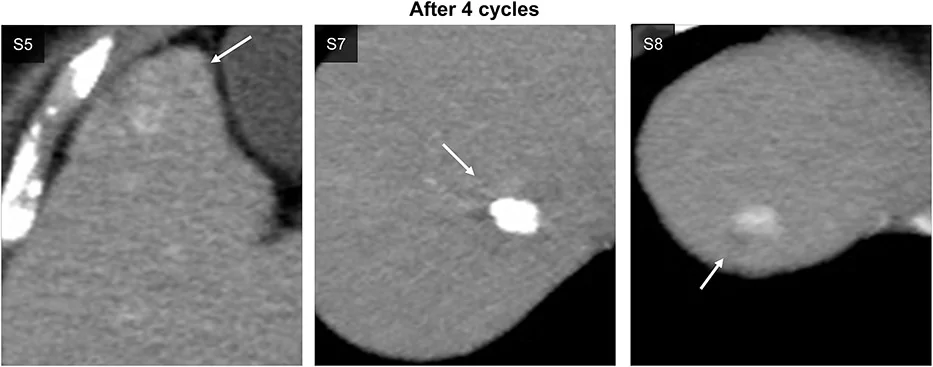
Arterial-phase CT conducted after 4 cycles of atezolizumab plus bevacizumab. The pre-existing lesions (segments 5 and 7) show no marked change, while a new intrahepatic lesion can be observed in segment 8 (arrows).
